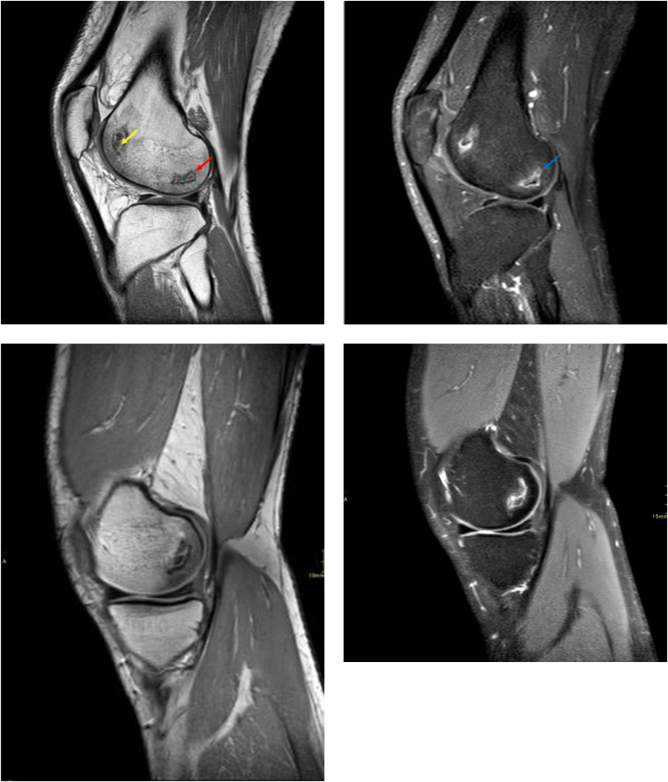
Sagittal T2-weighted image of the left knee shows geographic areas in the bone marrow of the lateral condyle of the distal femur, demonstrating the “double-line” sign, consisting of an outer sclerotic hypointense rim (red arrow) and inner hyperintense granulation tissue (yellow arrow), which is a pathognomonic MRI pattern seen at the periphery of an area of osteonecrosis, representing the boundary between viable and non-viable bone. Sagittal post-gadolinium fat-saturated T1-weighted image of the left knee shows contrast enhancement along the boundaries of the infarcted bone regions at the junction with viable bone tissue (blue arrow), and complete absence of contrast enhancement in the central necrotic area. Sagittal T2-weighted image of the right knee shows a geographic area in the bone marrow of the medial condyle of the distal femur, demonstrating the “double-line” sign, consisting of an outer sclerotic hypointense rim (red arrow) and an inner hyperintense granulation tissue (yellow arrow), which is a pathognomonic MRI pattern seen at the periphery of an area of osteonecrosis, representing the boundary between viable and non-viable bone. Sagittal fat-saturated proton-density image of the right knee redemonstrates the geographic area in the bone marrow of the medial condyle of the distal femur with abnormal increased signal intensity.
